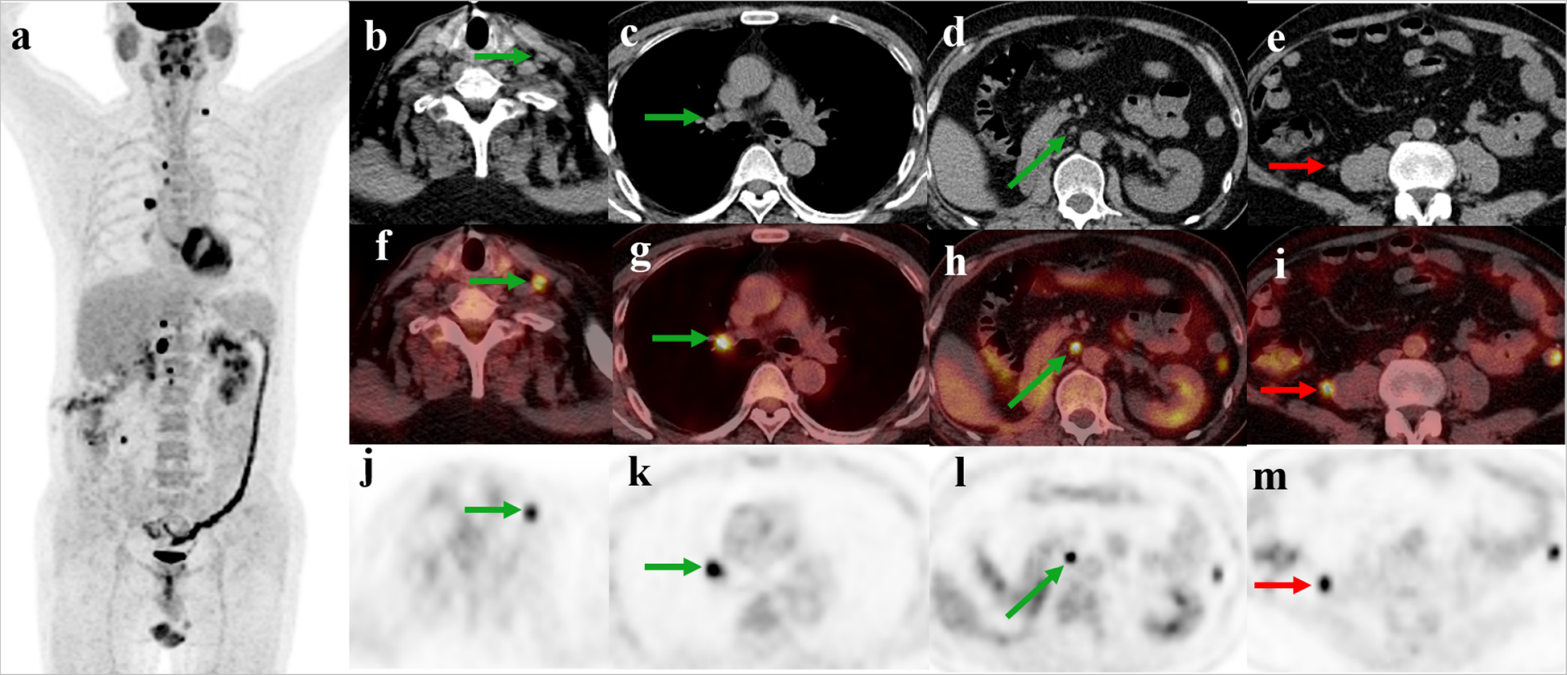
A 53-year-old man presented with multiple lymph node recurrences 3 months after right radical nephrectomy for renal cell carcinoma. Unenhanced diagnostic CT revealed enlarged lymph node in the retroperitoneum. FDG PET/CT (a-m) showed additional lymph node metastases (green arrows) in the left supraclavicular fossa, mediastinum, right hilum, retroperitoneum, and a hypermetabolic soft-tissue nodule (bule arrows) near the right psoas muscle, which were not large enough to be interpreted as positive at CT scan. Symmetric parotid gland uptake and post-surgical related uptake in the left renal region were also seen on PET/CT
